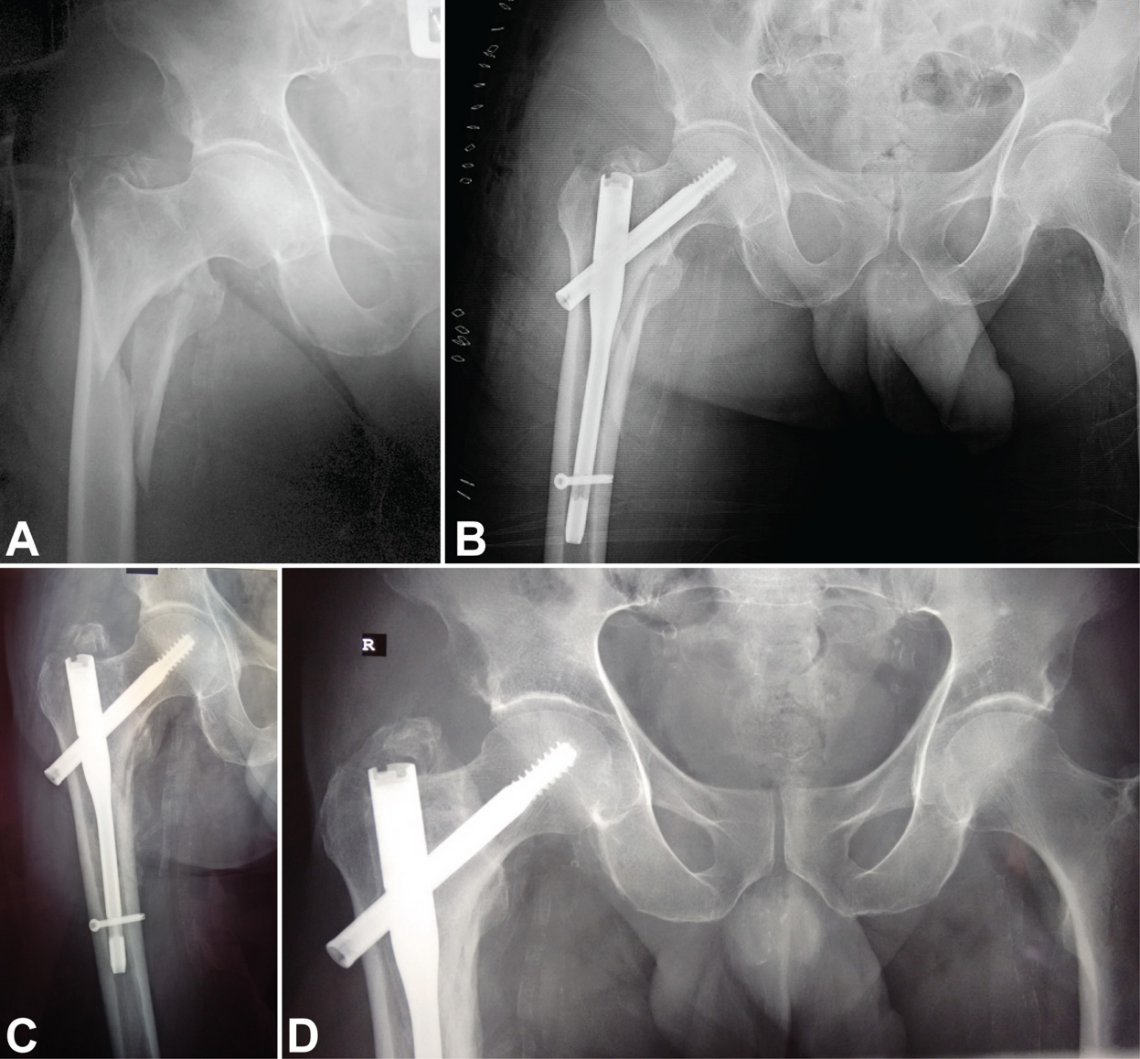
(A) Anteroposterior radiograph of the right hip of a 74-year-old man with an unstable pertrochanteric fracture (AO/OTA 31A2.2). (B) Postoperative radiograph after closed reduction and fracture fixation with a short intramedullary hip nail; the distance between the distal fracture line and the distal locking screw is 3 cm. (C) Anteroposterior radiograph of the right hip at 3-month follow-up shows fracture healing; the patient was weight-bearing with a cane. (D) Anteroposterior radiograph of the right hip at 12-month follow-up shows complete fracture healing.
